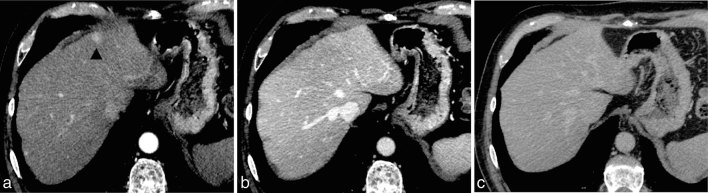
(a) A contrast-enhanced axial CT image acquired during the hepatic arterial phase demonstrates an enhancing lesion measuring approximately 14 mm in the left lobe of the liver (black arrowhead), which shows isodensity to the surrounding parenchyma in the images acquired during the portal venous phase (b) and the delayed phase (c).
